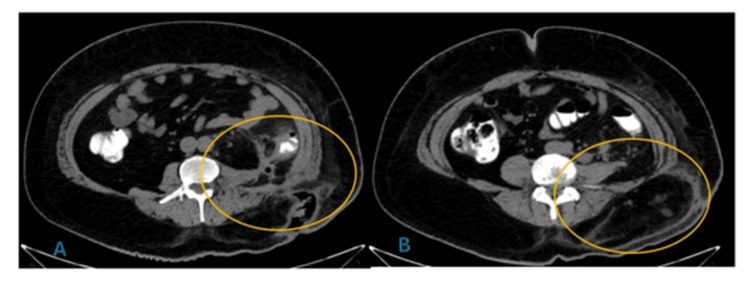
Axial abdominal CT scan with water-soluble contrast administered rectally. (A) Yellow markings indicating a fibrotic band retracting the descending colon and left psoas muscle extending into the fistulous tract, with no extravasation of contrast medium into the abdominal cavity. (B) Saccular image at the junction of the descending colon and sigmoid colon protruding beyond the wall.
